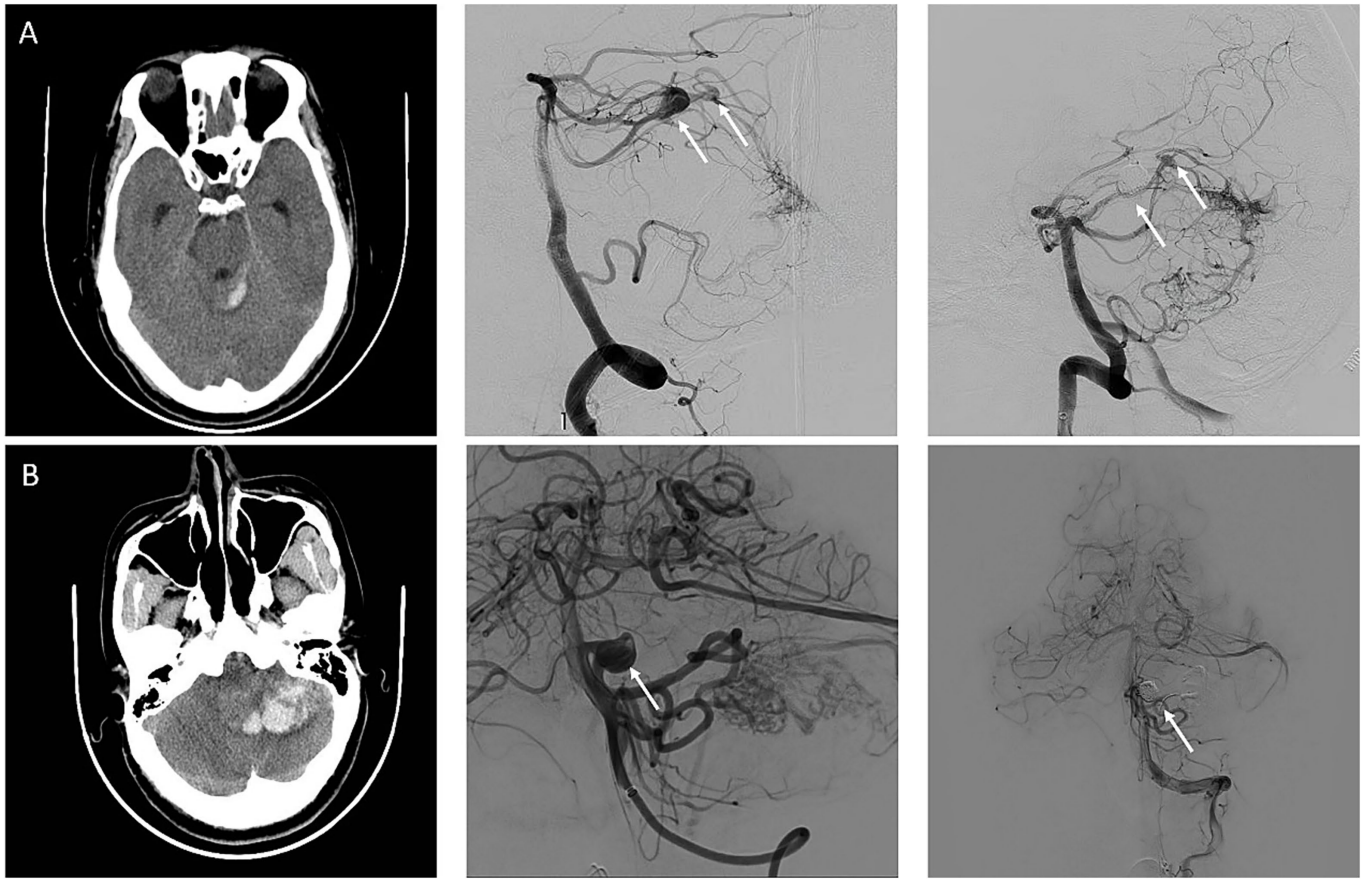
Representative cases of ruptured flow-related aneurysm and unruptured flow-related aneurysm. (A) An adult patient with a sudden headache was diagnosed with subarachnoid hemorrhage on a computed tomography (CT). Arteriovenous malformation fed by the left superior cerebellar artery and two flow-related aneurysms (white arrow) were detected on digital subtraction angiography. The larger FAs with a maximum diameter of 10 mm were the reason for hemorrhage and was treated with coils for the first time. (B) An adult patient diagnosed with intracerebral hemorrhage due to immediate headache and imbalance by CT. AVMs fed by the left anterior inferior cerebellar artery and posterior inferior cerebellar artery and FAs (white arrow) located in the anterior inferior cerebellar artery were detected in DSA. FAs and AVMs were treated with coils and onyx at the same time.
